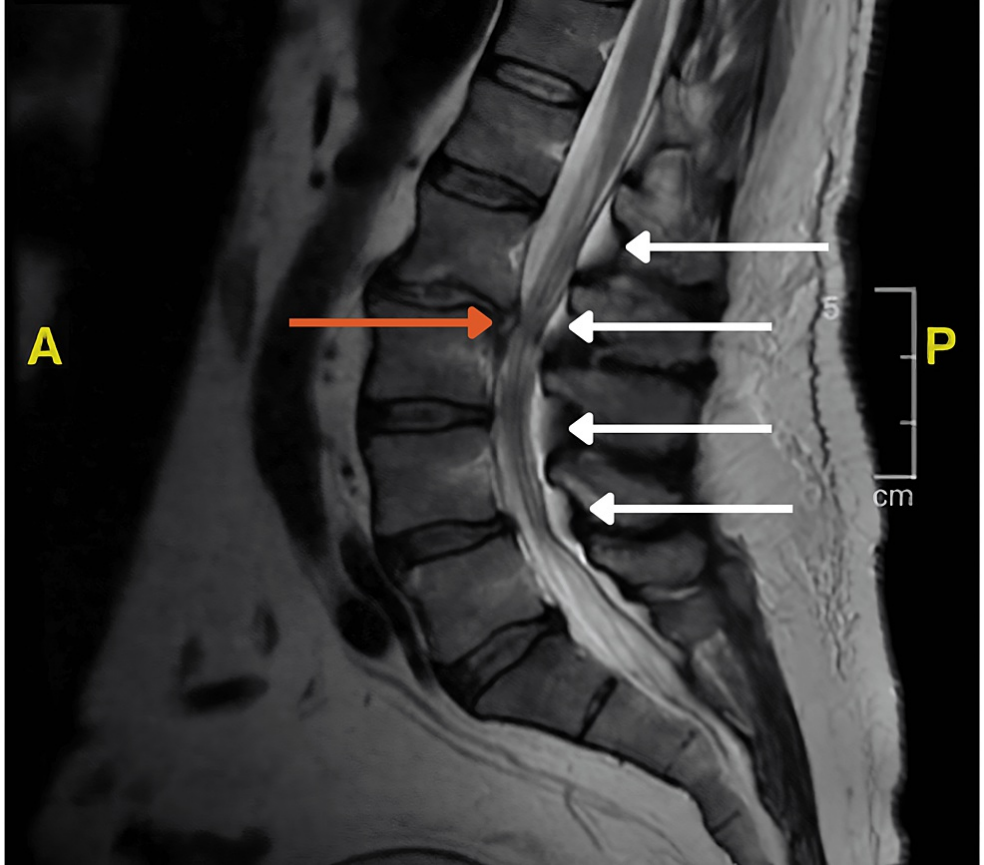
MRI T2 with contrast of the lumbosacral spinal cord showing fat compression at multiple levels (white arrows). L2-L3 disc herniation (orange arrow).
